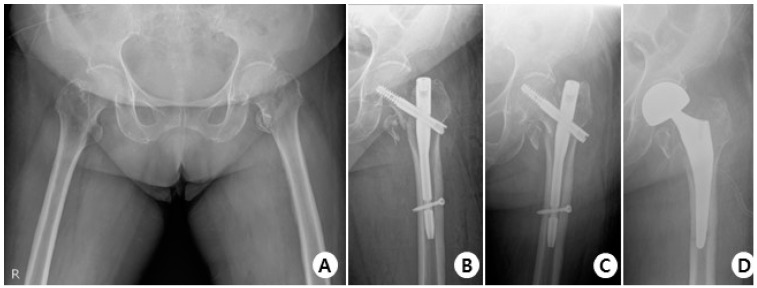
(A) Total hip AP X-ray of a 76-year-old female diagnosed with an intertrochanteric fracture of the left hip. (B) Immediately post-operation, the fractured segment was reduced in a slight valgus position using the GS hip nail implant. (C) The two months postoperative X-ray shows reduction loss and the progression of varus deformity, with the lag screw experiencing cut-out. (D) X-rays show the revision surgery performed with arthroplasty.
